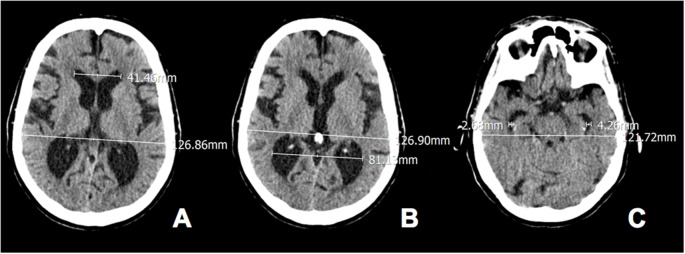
Calculated Evan’s index, parieto-occipital ratio (POR) and temporal ratio (TR) in axial computed tomography (CT) scans of a 80-year old woman. (A) Evans’ index (EI) 41.46/126.86 = 0.33; (B) POR 81.13/126.90 = 0.64 and (C) TR 2.68 + 4.26/121.72 = 0.06.
